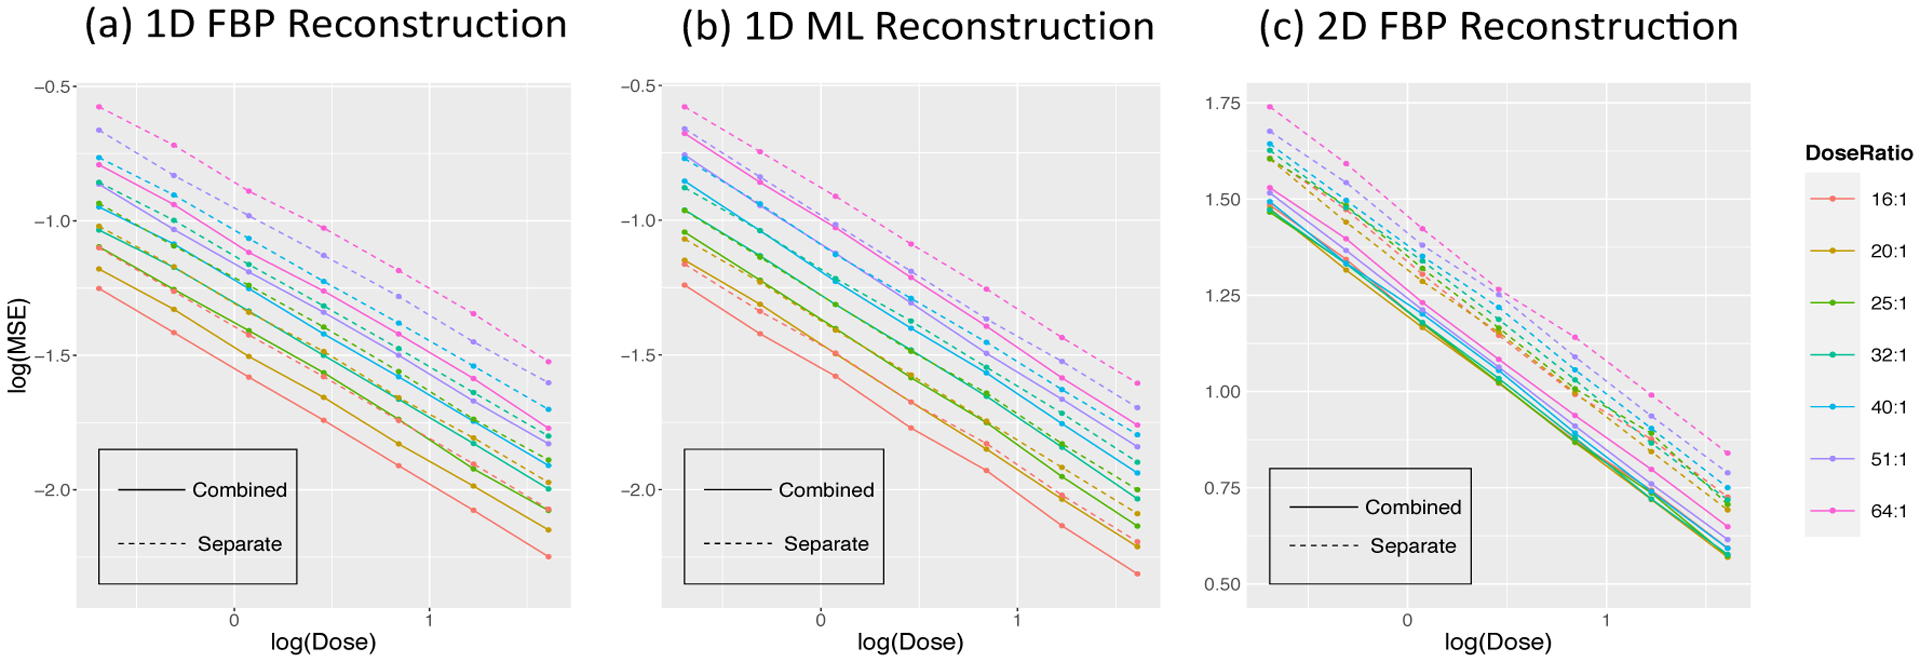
MSE of combined (solid) and separate (dashed) analysis as a function of dose and dose ratio in H_2_O-FDG study. Different colors represent different dose ratios.
